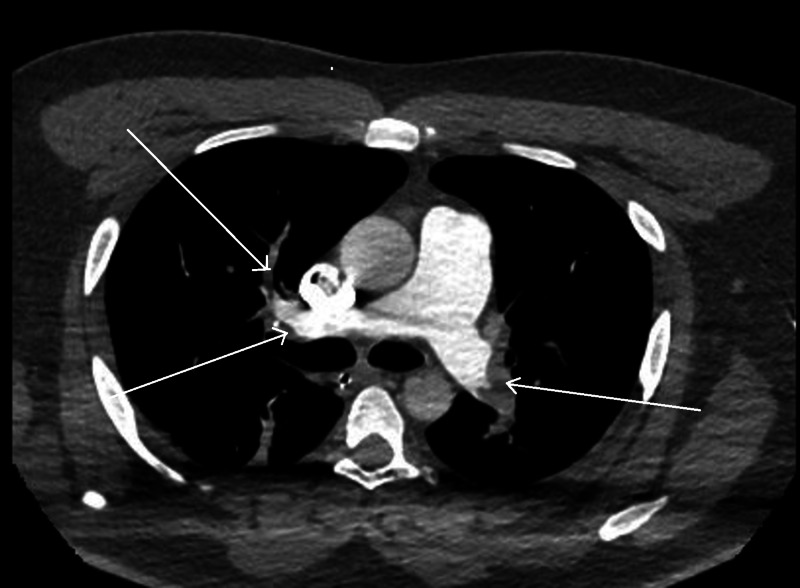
Computed Tomography –Angiography (CT-PA) of the chest, mediastinal window Large filling defects in the main, segmental, and subsegmental branches of pulmonary arteries
